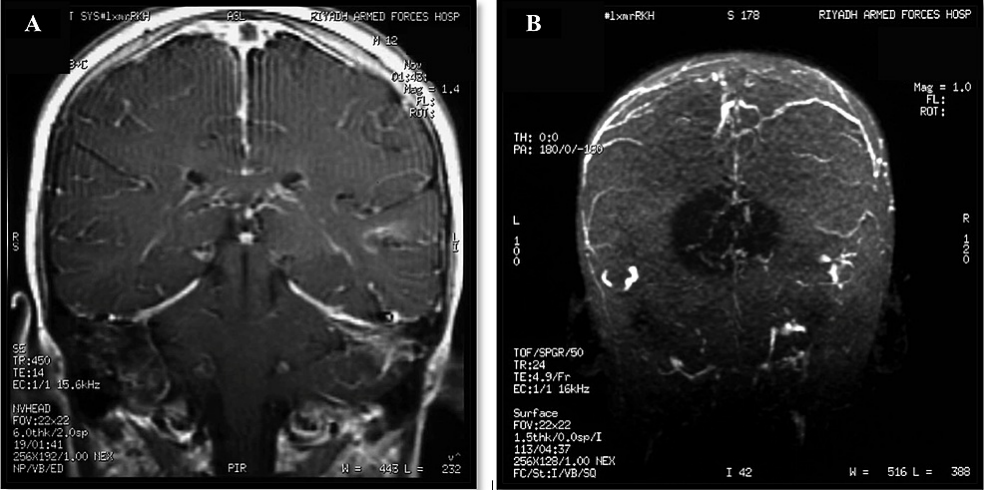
Patient's MRI and MRV showing: (A) coronal T1 post-contrast venogram of the brain demonstrate non-opacification of the superior sagittal sinus with empty delta sign as well as the transverse sinuses. (B) Single MIP image from a 3D SPGR post-contrast demonstrating non-visualisation of the superior sagittal sinus, inferior sagittal, and transverse sinuses. MRI: magnetic resonance imaging, MRV: magnetic resonance venography, MIP: maximum intensity projection, 3D SPGR: three-dimensional spoiled gradient recalled echo, T1: longitudinal relaxation time.
